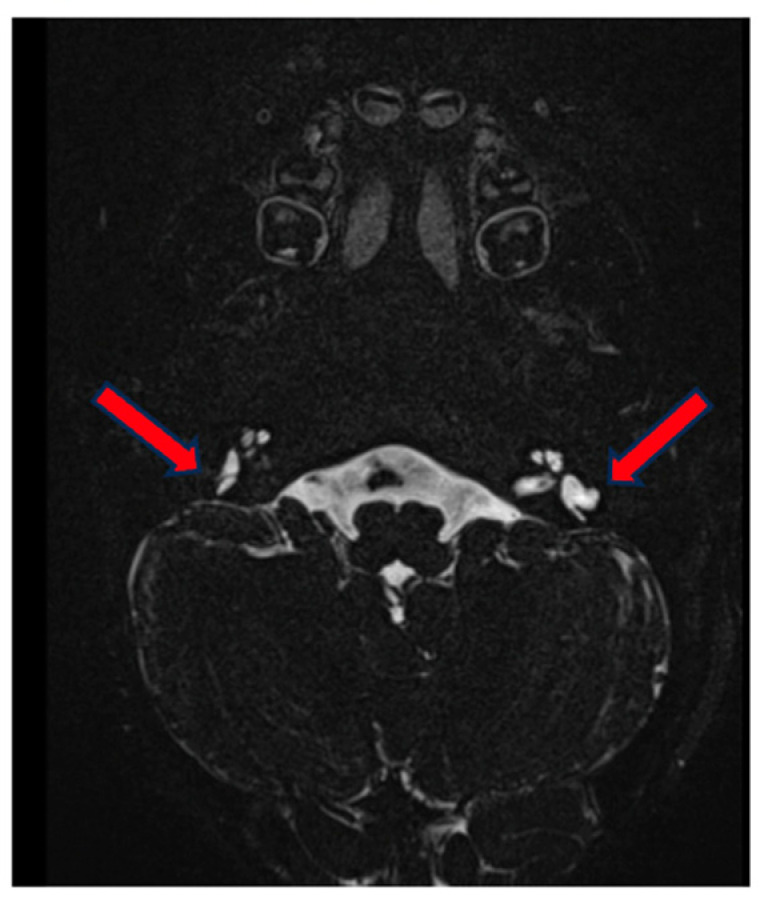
MRI of the inner ear for case 2, T2 tse3d weighted image, dysplasia of lateral semicircular canals (red arrows).
